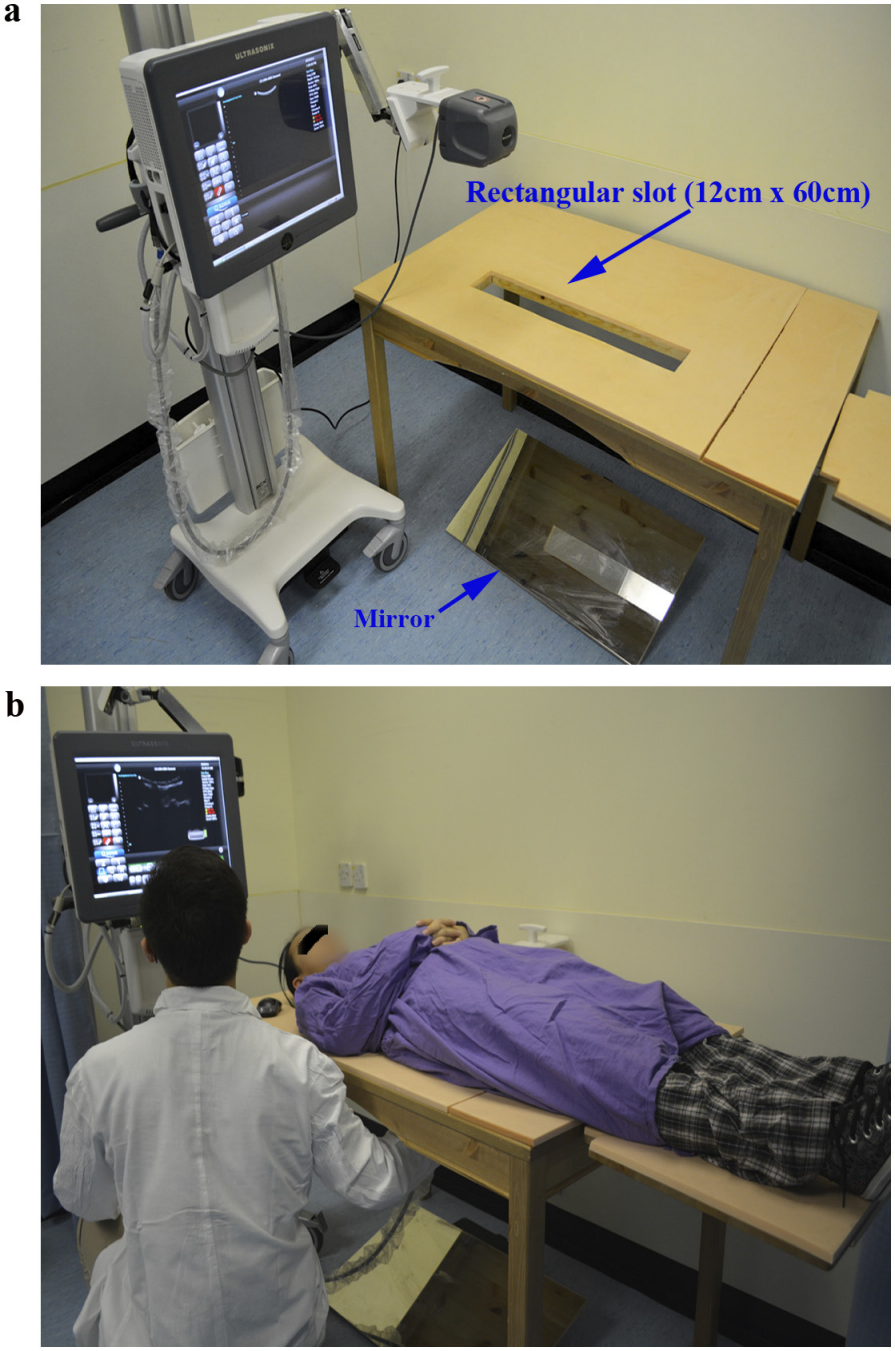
Clinical ultrasound system and ultrasound scan. (a) 3-D Ultrasound unit and a purpose-design couch; (b) Ultrasound scanning at supine position.
